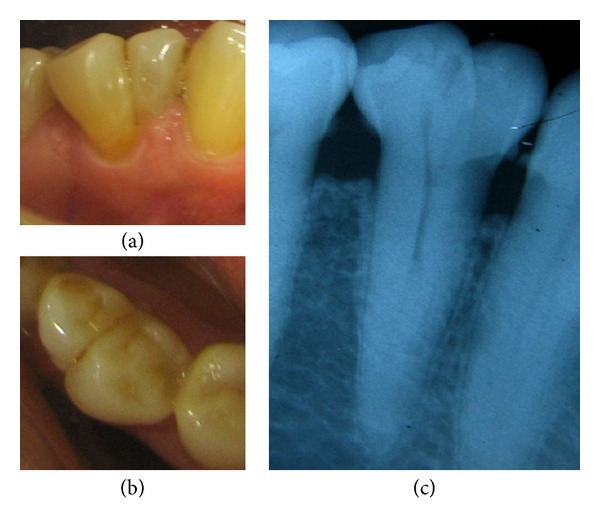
Photographs (a and b) and multiple angulated radiographs (c) of a geminated mandibular first premolar showing the complexity of the internal and external and coronal and radicular anatomy. Multiple canals that fuse and redivide add to the endodontic challenge.
